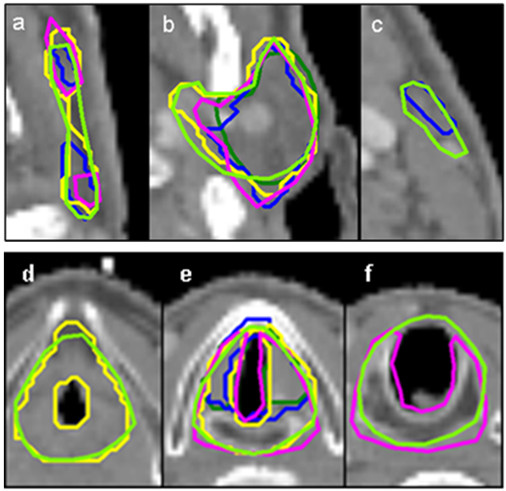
Delineation variation of a parotid gland and a glottic larynx. Left parotid gland and glottic larynx delineations in a typical cranial (a and d), central (b and e) and caudal (c and f) transverse CT slice. Each colour corresponds to one observer.
